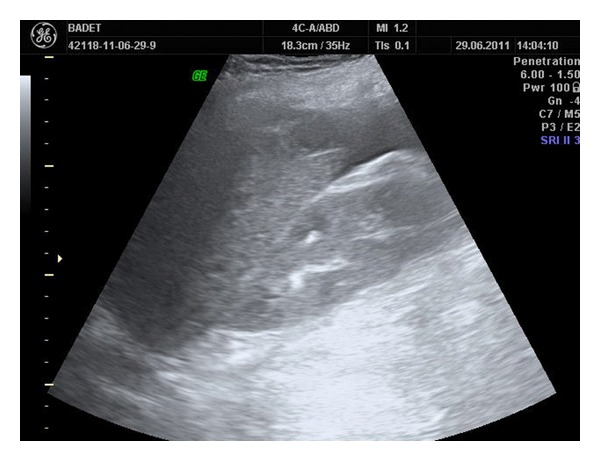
Liver ultrasound after 15 minutes reveals growth of the subcapsular hematoma.
